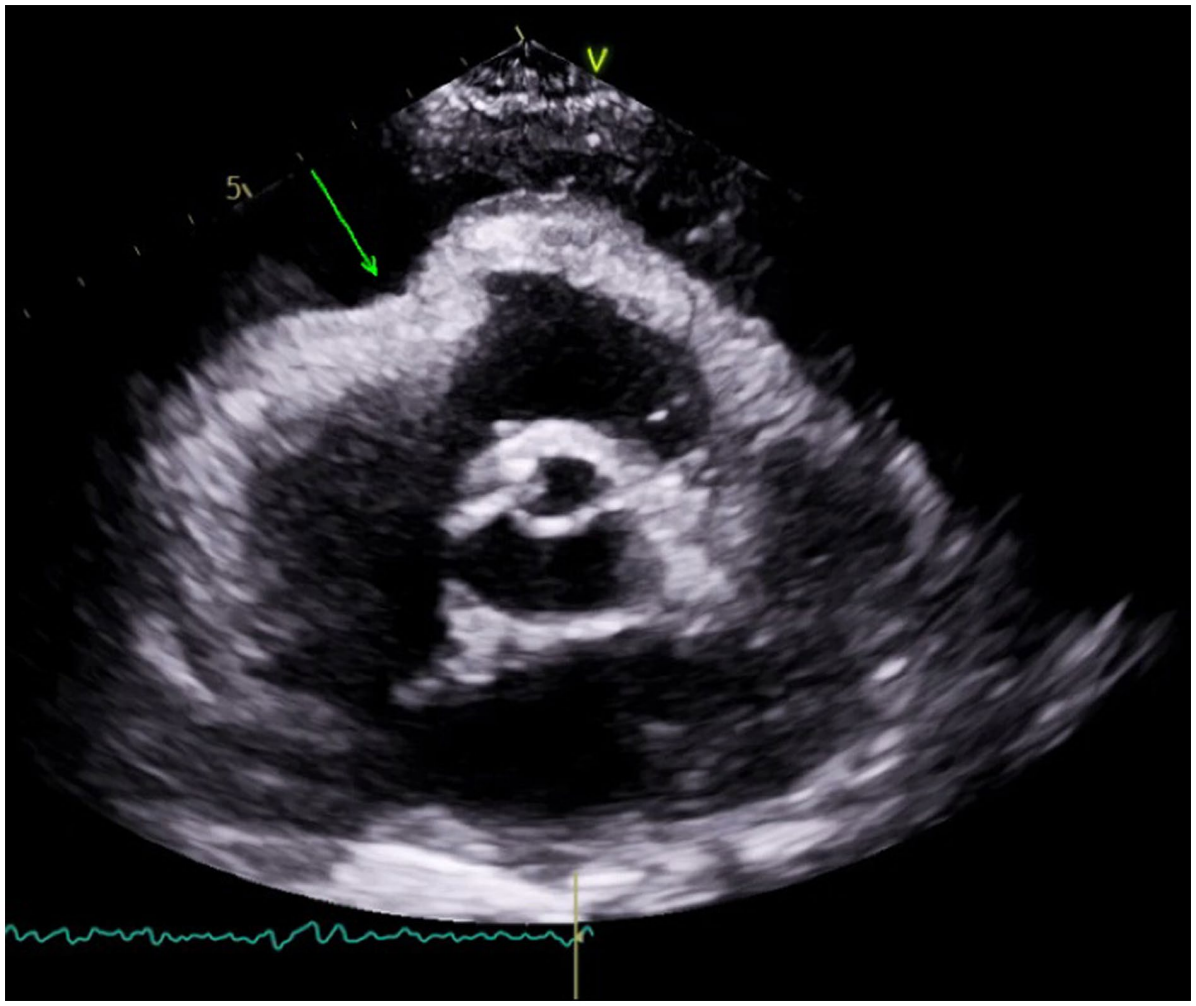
Parasternal short axis view showing pericardial effusion with RV collapse.
